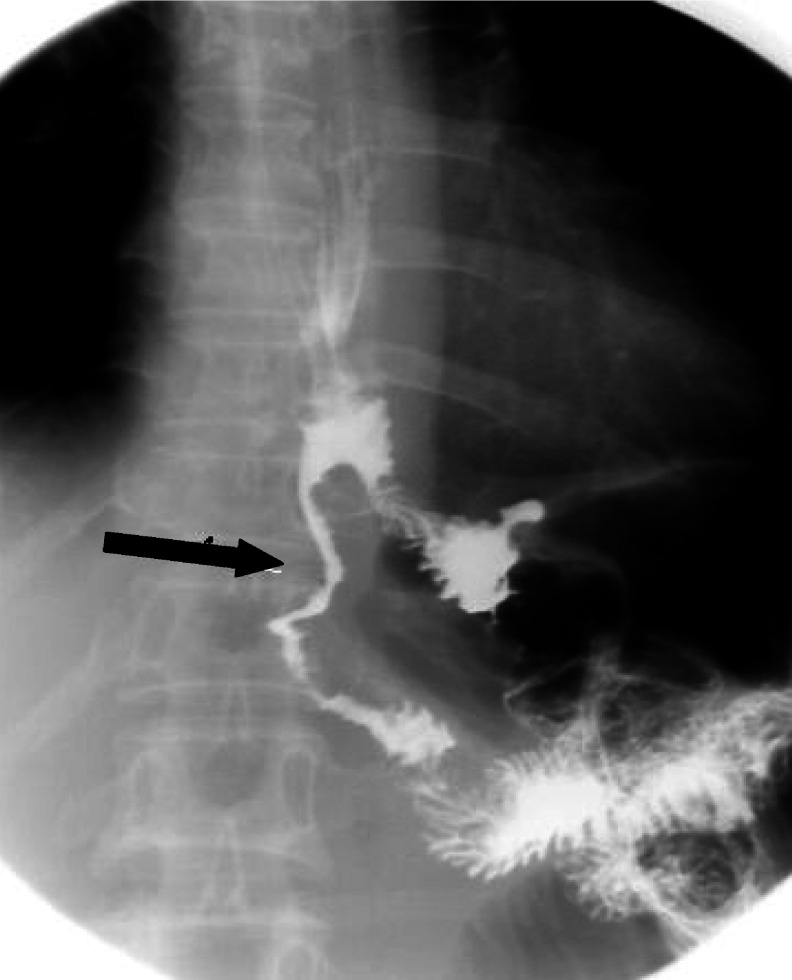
Upper gastrointestinal radiograph of a patient who developed intestinal radiation fibrosis. The black arrow indicates segmental narrowing of the jejunal loop below the anastomosis site.
